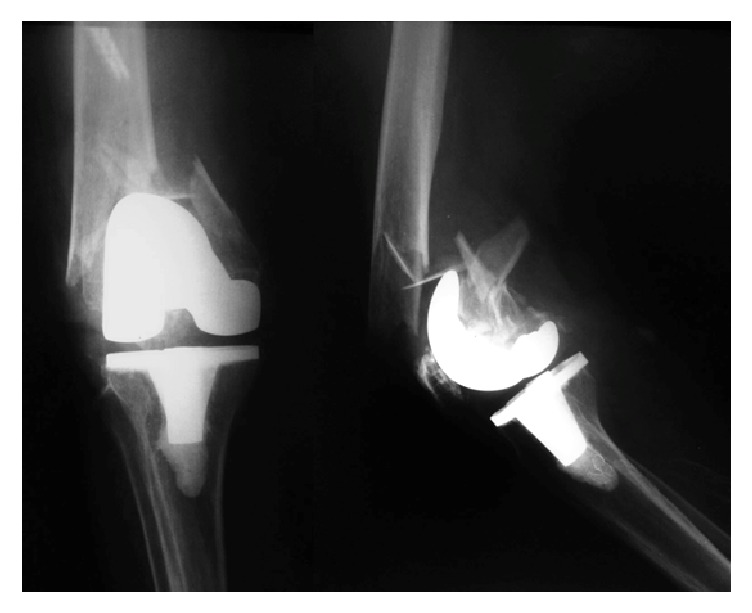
Presenting knee injury X-rays. Note the severe comminution of the metaphyseal area, including the medial wall of the distal femur. Radiologically, the femoral component seemed to be fixed.
